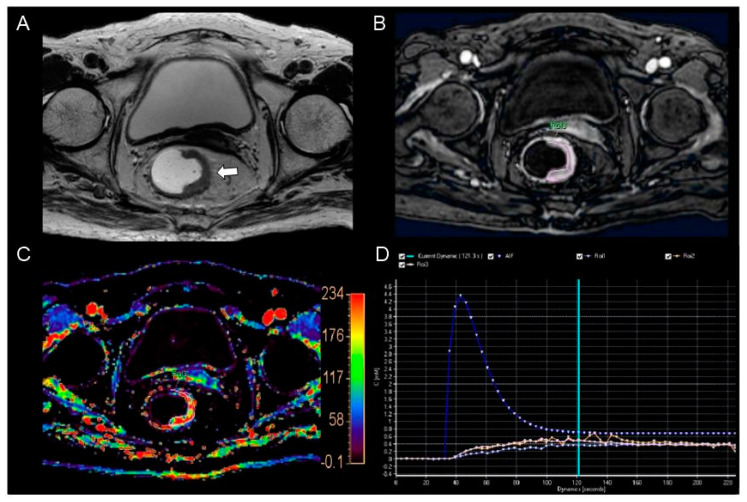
MR images from an 86−year−old woman with pathologically proven well−differentiated rectal adenocarcinoma. (A) The T2−weighted axial image shows an ulcero-infiltrative mass (arrow) in the left wall of the mid rectum. (B) The post−contrast axial 3−dimensional spoiled gradient echo image shows a relatively hyper−enhancement in the corresponding tumor. (C) The color−coded K^trans^ map shows a predominantly red color in the corresponding tumor. The calculated mean K^trans^ was 0.137 min^−1^. (D) The concentration time curve shows a plot of arterial input function (AIF, blue line), which was incorporated automatically with the population−based data and three plots from the consecutive three regions of interest (ROIs−1, 2, and 3) in the whole tumor (pale blue and orange lines).
